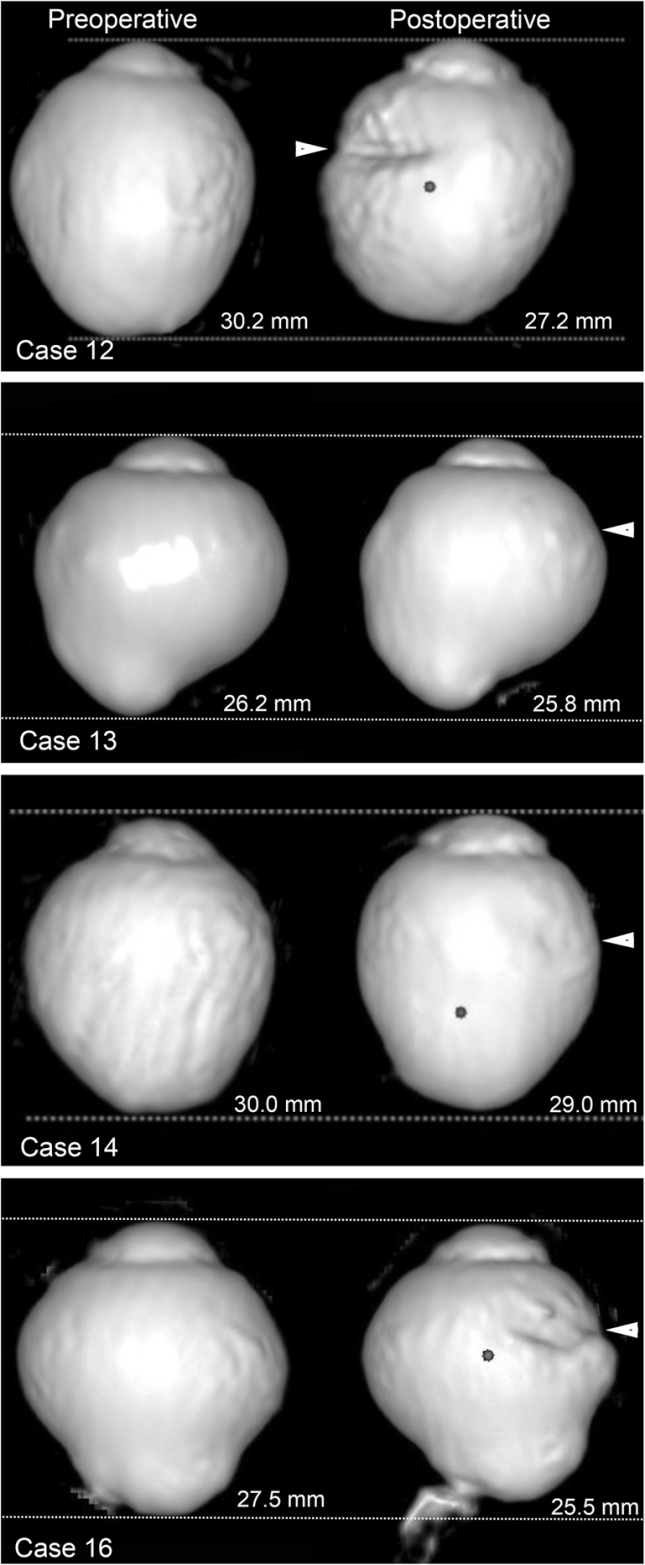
Preoperative and postoperative three-dimensional magnetic resonance imaging (3D-MRI) images. 3D-MRI images show posterior extrusion of the eye preoperatively in all eyes. The postoperative images show the infolding caused by the scleral imbrication (*white arrowheads*) and the decrease of the axial length of the eye. The curvature of the posterior surface of the staphyloma became less extruded posteriorly after surgery
